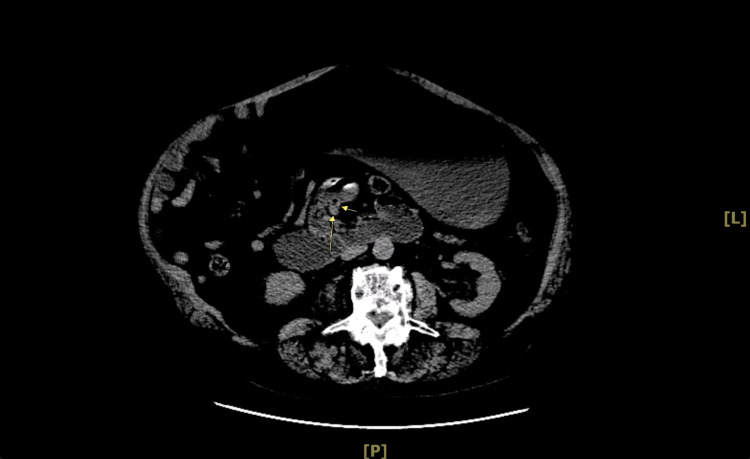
Abdominopelvic CT scan without contrast results. Yellow arrows: The whirl-like appearance of the bowel around the SMA. SMA, superior mesenteric artery; CT, computed tomography
